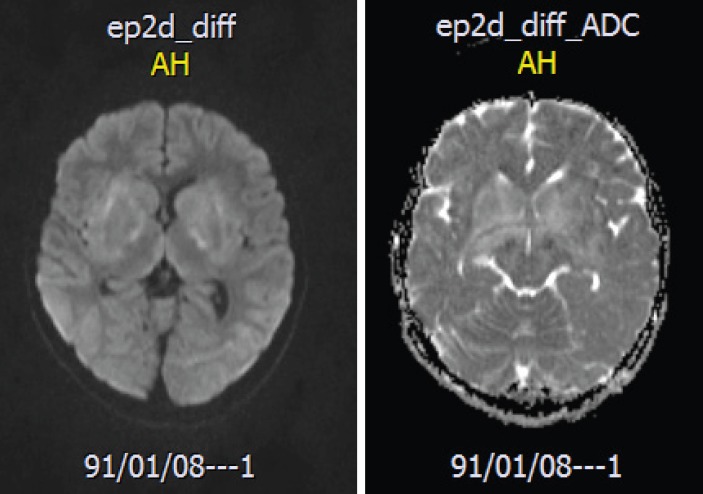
Brain MRI image DWI and ADC map showing no restricted
